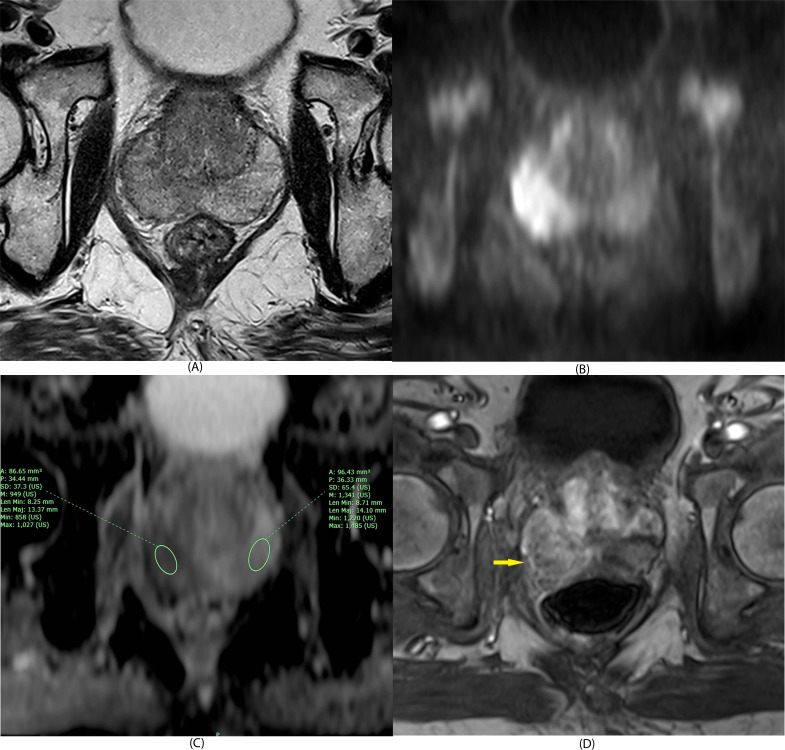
A 65-year-old patient with a target lesion located at the right peripheral zone midgland level. Axial T2-weighted image (A), diffusion-weighted image (B), ADC map with measurement (C), and axial contrast-enhanced image (D) are shown (yellow arrow). ADC: apparent diffusion coefficient.
